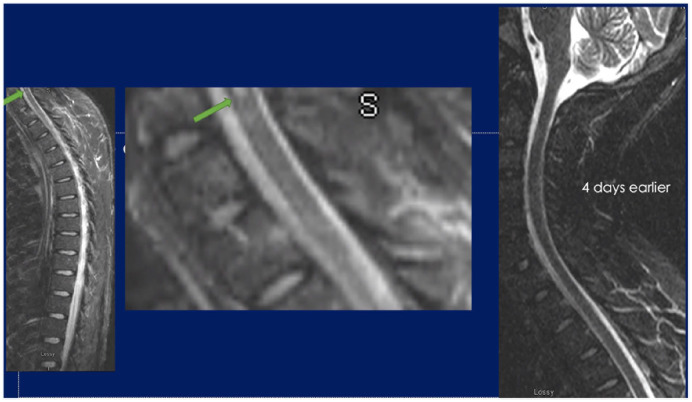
MRI revealing rhombencephalitis with displayed increased FLAIR and T2 signals involving the lower brainstem at the level of cerebellar peduncles and upper cerebellum in symmetric fashion compared to initial MRI at admission (right).
